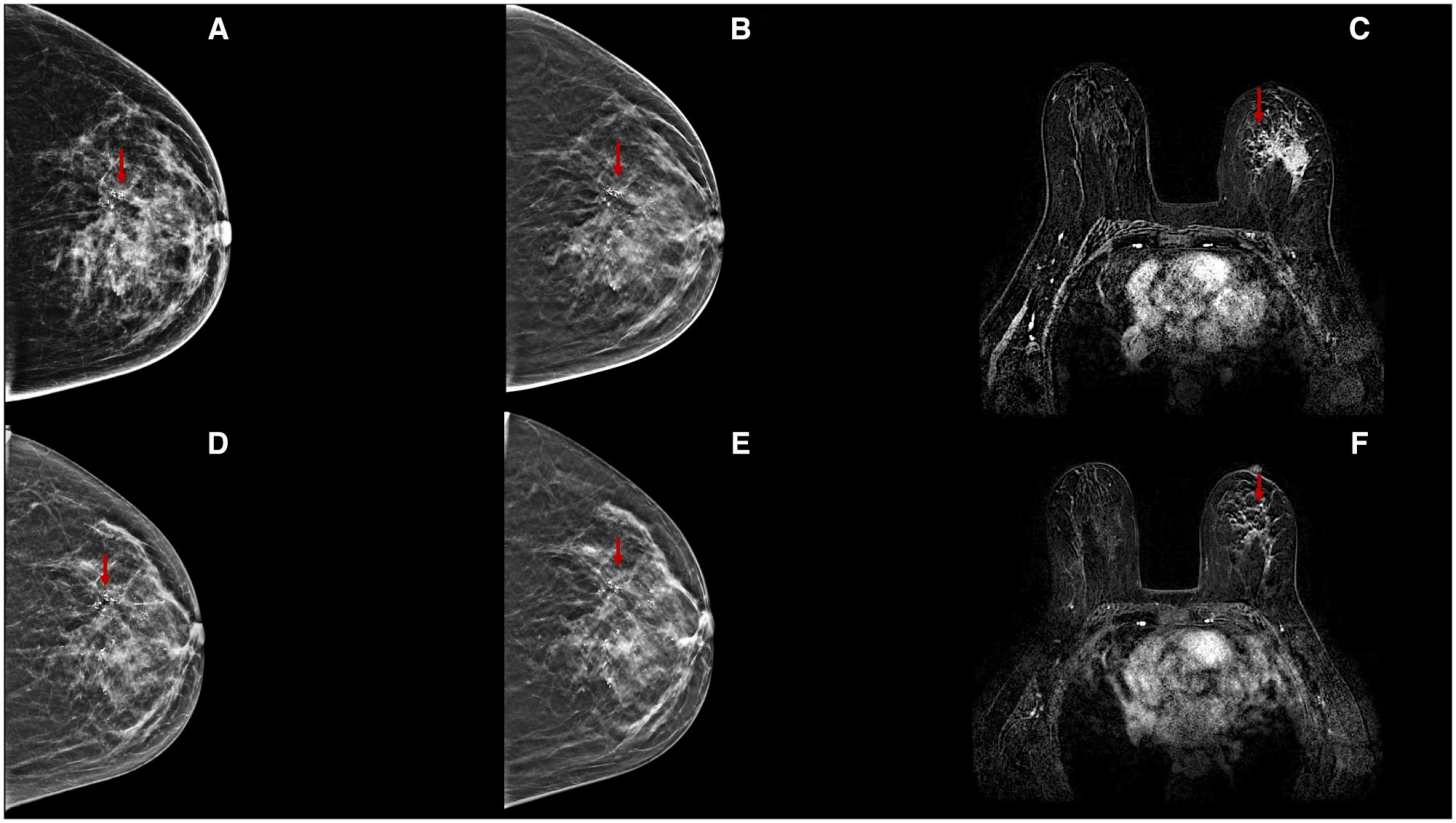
A 44-year-old woman underwent NT (four cycles of epirubicin-cyclophosphamide followed by weekly trastuzumab + paclitaxel for 12 weeks) for an invasive ductal carcinoma cT2cN0, G3 (ER 40%, PgR 0%, Ki-67 25%, HER2 3+). Cranio-caudal DBT before (B) and following (E) NT better highlight an extensive area of a pleomorphic microcalcification with increased parenchymal density in the upper half of the left breast (arrows), compared to DM (A, D). At the end of therapy, DBT allows a better assessment of the areas of radiolucency for concentric shrinkage, confirmed on MRI subtraction image (F).
